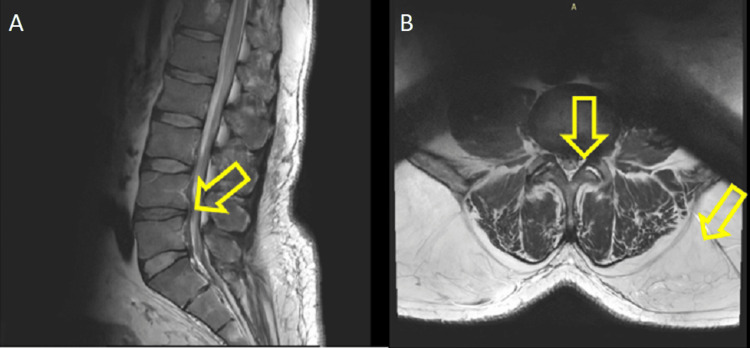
MRI: (A) Sagittal plane, MRI of the lumbar spine discovered dehydration and degeneration of the disc of L4-L5, narrowing of the disc space of L4-L5, and (B) axial plane, L4-L5 foraminal disc herniation
